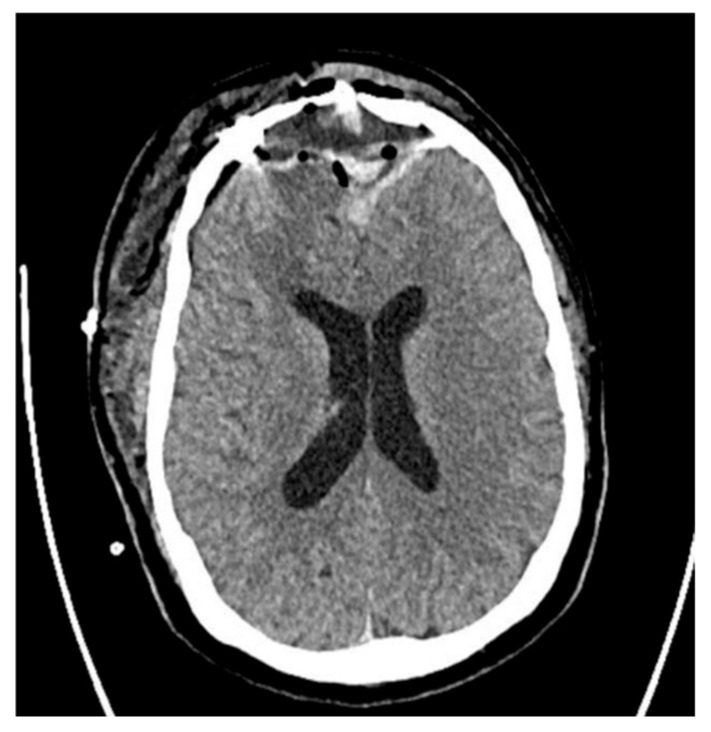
Postoperative head CT showing resolution of brain edema, implanted drains, and the bone graft.
